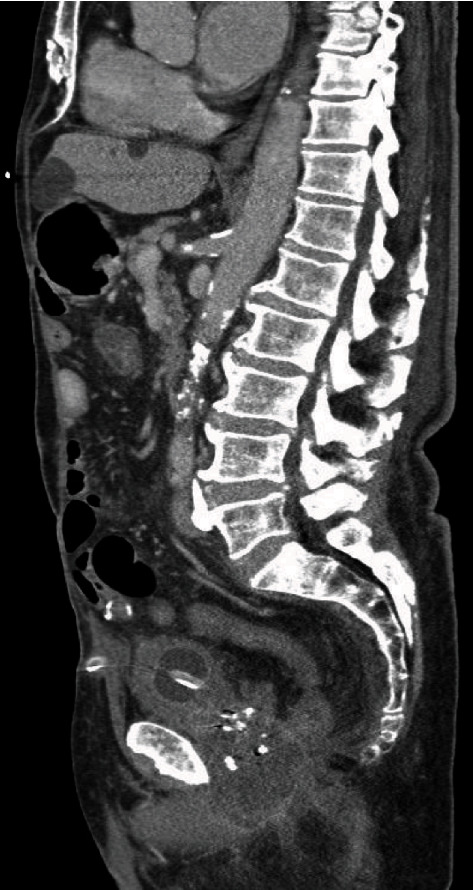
Saggital CT imaging demonstrating persistence of collection post antibiotic treatment.
